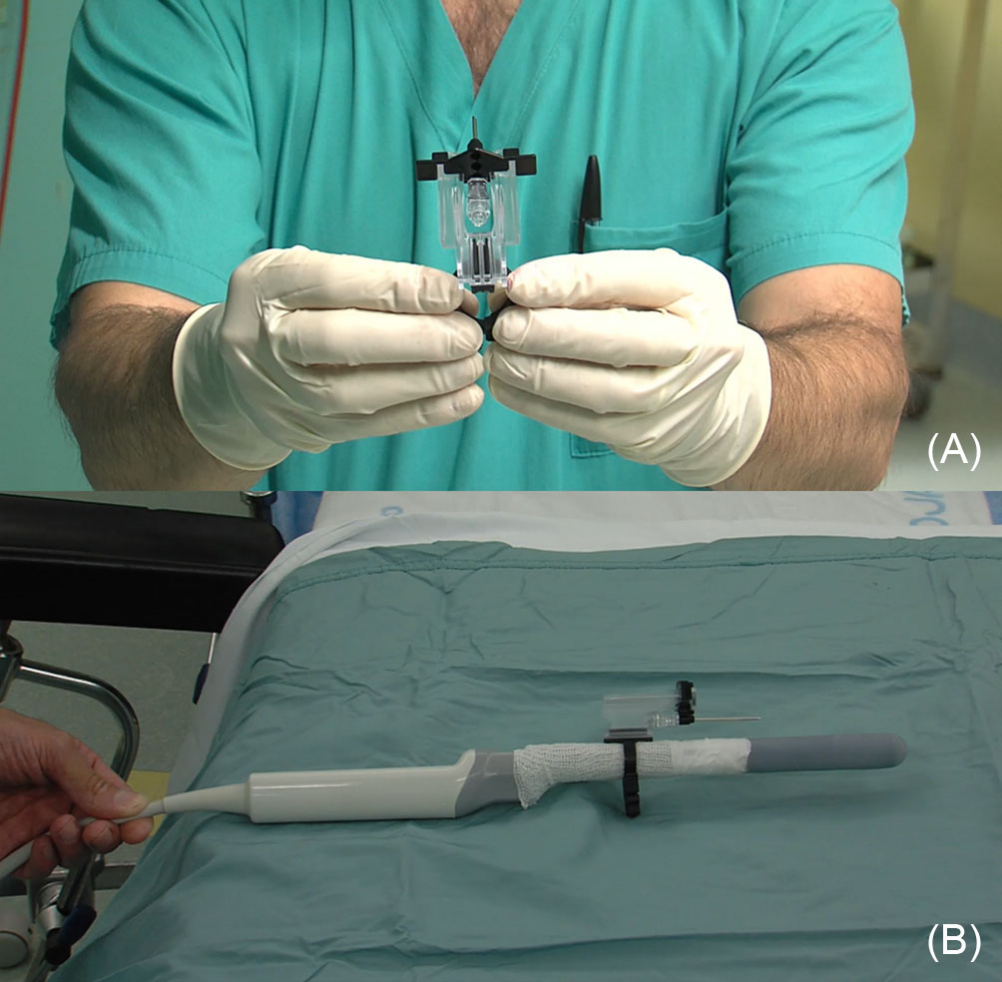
(A) Frontal view of the three components of the PrecisionPoint Transperineal Access System after assembly, with the 15 G perineal access cannula inserted into the lowermost of the apertures of the needle carriage. (B) Tethering of the device to a biplanar transrectal ultrasound probe; proper positioning ensures that the tip of the 15 G cannula intercepts the part of the beam emitted by the linear transducer that is closest to the operator. Padding made from cohesive bandage ensures adequate compatibility of the clamp/rail subassembly with probes of non‐circular cross‐sections.
